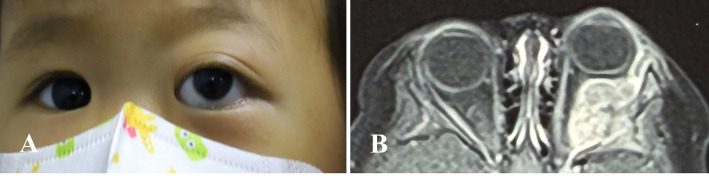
Initial appearance and radiographic findings. A Clinical photograph showing left proptosis. B Contrast-enhanced MRI demonstrating a retrobulbar mass in the left lateral orbit extending toward the orbital apex, with focal bone erosion
